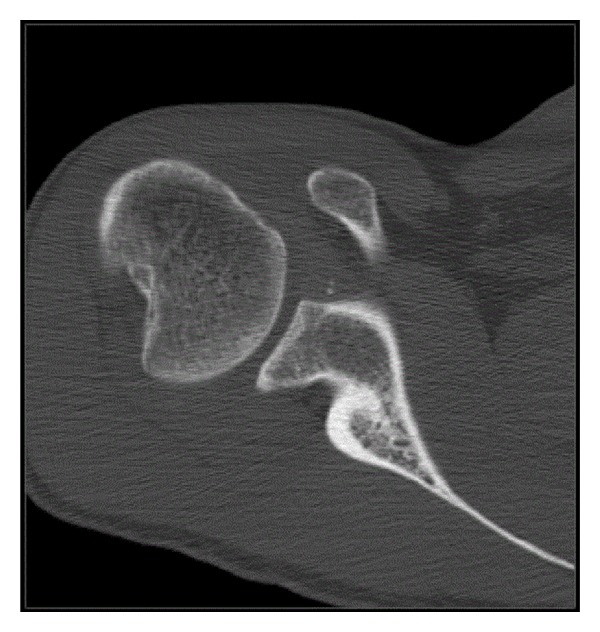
CT scan which demonstrates a large Hill-Sachs lesion.
